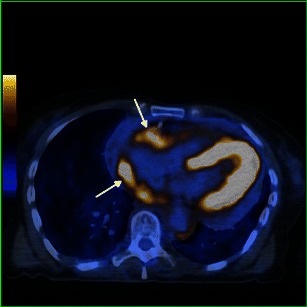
Axial FDG-PET/CT fusion images in a 71-year-old female, showing pericardial and epicardial thickening, with elevated metabolic activity, namely in the right atrium and atrio-ventricular sulcus (arrows)
